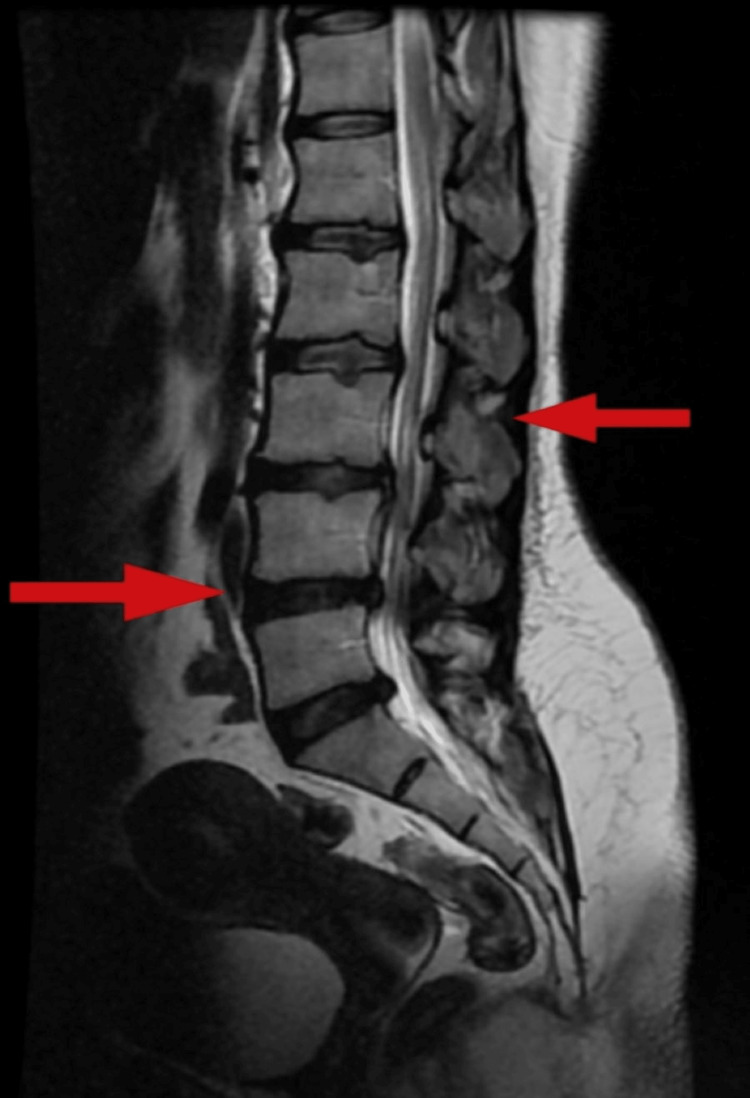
Pre-operative MRI of the spine showing stenosis at level of L3-S1 (red arrow) MRI - magnetic resonance imaging
